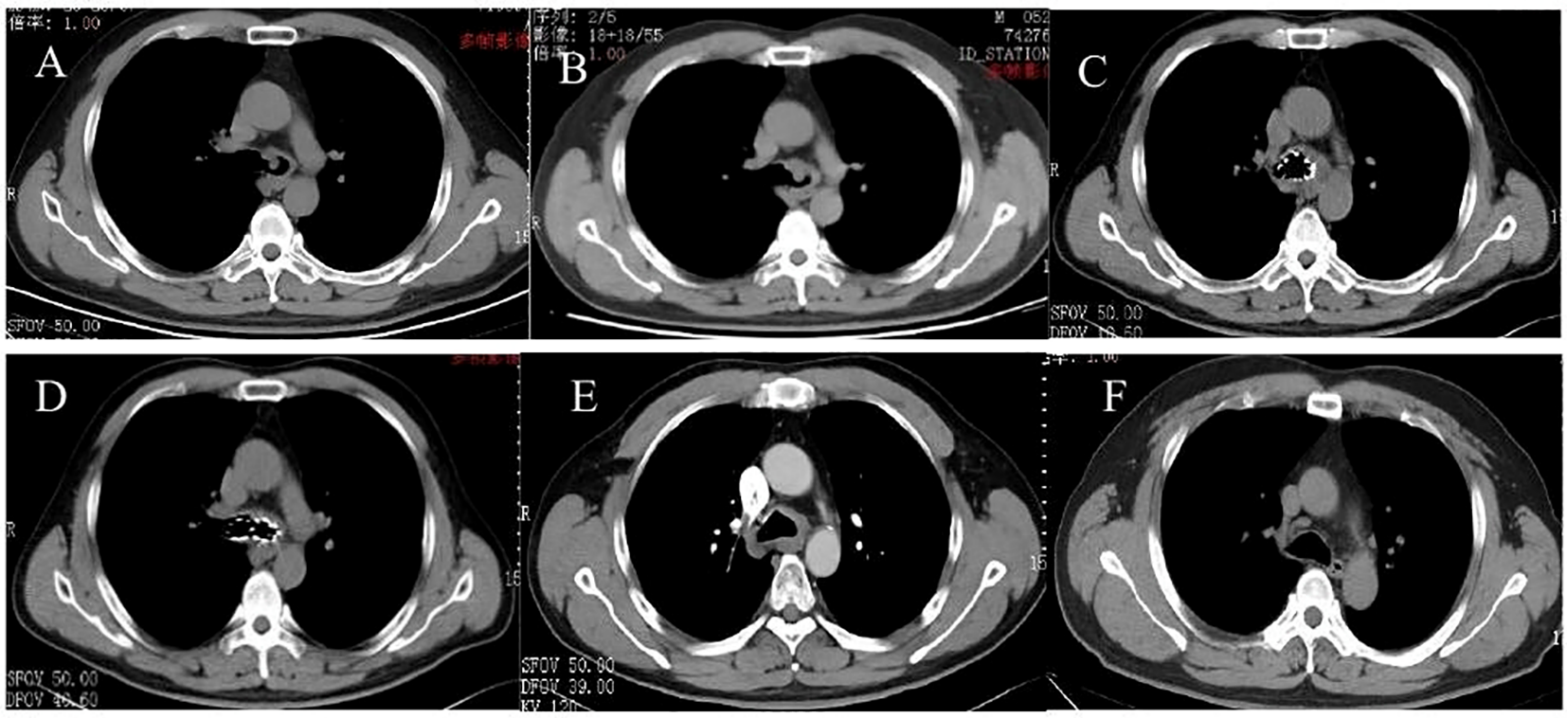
CT pictures. (A) 5 January 2017, tracheal adenoid cystic carcinoma (TACC) on the left side of the lower trachea near the carina. (B) 5 January 2019, TACC recurred. (C, D) 18 April 2019, ^125^I seed stent implanting. (E) 21 June 2019, after the ^125^I seed stent removing. (F) 17 August 2023, the main bronchus more than 4 years after removing the stent.
